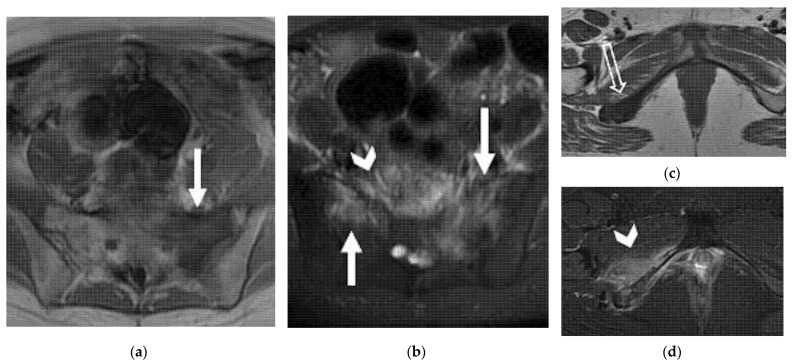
(a,b) A 56-year-old patient who underwent radical hysterectomy with BSO and chemoradiation therapy for cervical cancer. (a) Axial FSE T1 weighted and (b) axial FSE T2-weighted fat-sat images show osseous metastases in the sacrum, characterized by hypointense T1 and hyperintense T2 signal intensity (solid arrows). (c,d) A 41-year-old patient who underwent previous chemoradiation therapy for cervical cancer. (c) Axial FSE T1 weighted and (d) axial FSE T2-weighted fat-sat images show osseous metastases in the ischio–pubic right branch (open arrow in c). Note the oedema in the adjacent soft tissue and muscles (arrowheads in (b,d)).
